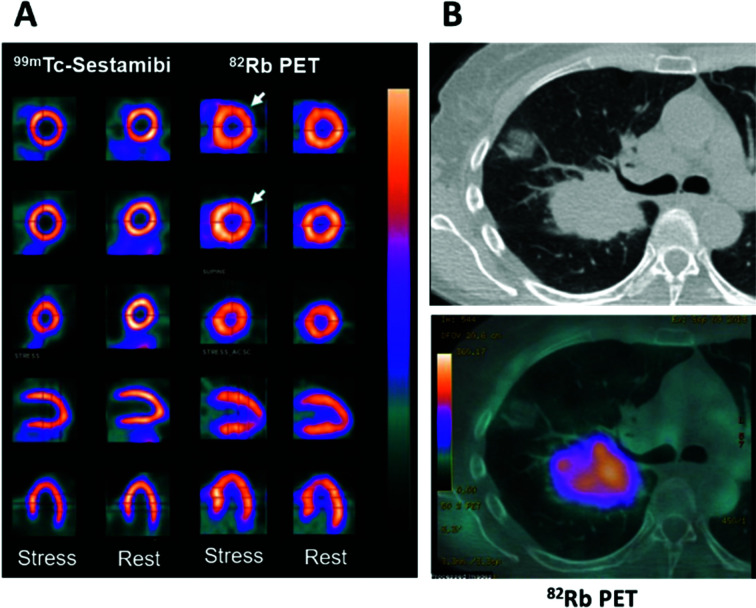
Applications of potassium mimetics in nuclear medicine. (A) Representative myocardial perfusion imaging with ^99m^Tc-Sestamibi SPECT and ^82^Rb-PET.^14^ From top to bottom: axial apical, mid and basal sections, coronal and sagittal slices. (B) Clinical CT scan of a lung cancer patient (top) with a corresponding axial section from a ^82^Rb PET scan (bottom) (courtesy of A. Groves, UCL). Myocardial perfusion images are adapted and reproduced with permission under a Creative Commons Attribution (CC-BY) License from ref. 14, *Front. Med*., copyright© 2015.
